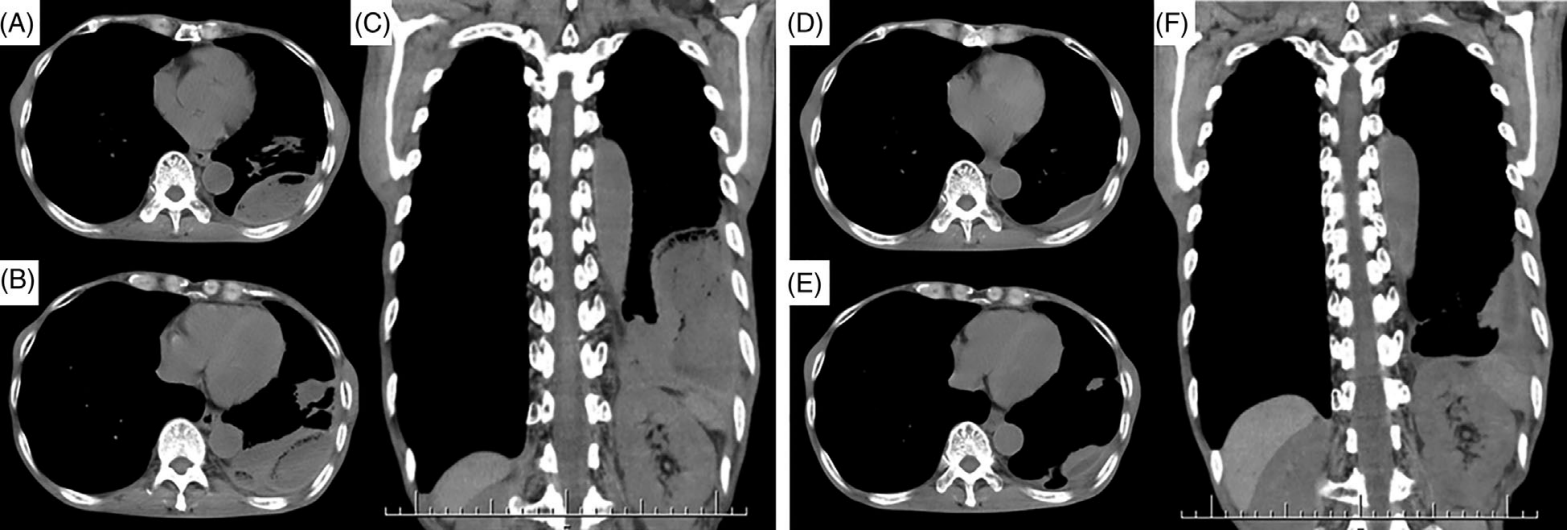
Computed tomography of images. (A–C) Before treatment, chest computed tomography (CT) revealed suspended air bubbles within the pleural effusion with septations in the left lower lung lobe. (D–F) After therapeutic thoracentesis and treatment with antibiotics for 4 weeks, CT showed marked shrinkage of the empyema, and no septations or gas foci were detected in the pleural effusion
